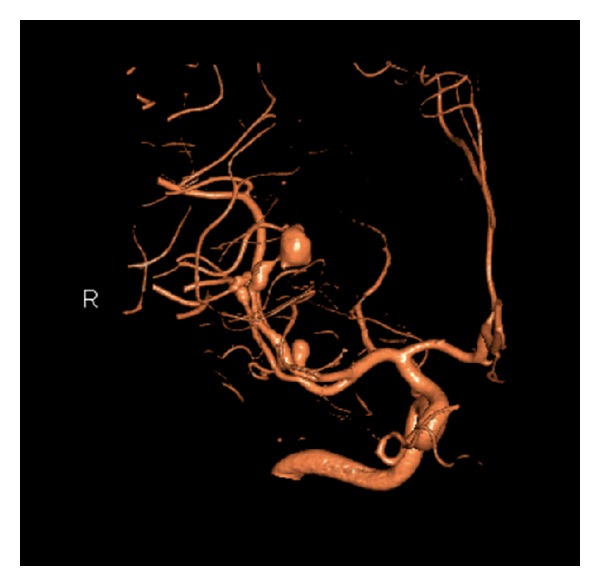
Oblique CTA scans demonstrating an ectatic fusiform dilatation of the involved MCA distal branch with aneurysm, in addition to saccular smaller aneurysm at the origin of MCA bifurcation.
